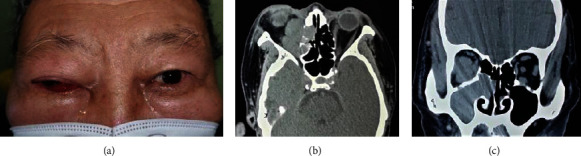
(a) Clinical photograph of a 76-year-old man with orbital metastasis of lung cancer showed ptosis, eyelid swelling, and chemosis of the right eye. (b) The coronal computed tomography showed a massive mass replacing the medial aspect of the orbit and ethmid sinus. (c) The coronal computed tomography scan showed an irregular mass in the right orbit replacing the orbit, ethmid sinus, and maxillary sinus.
